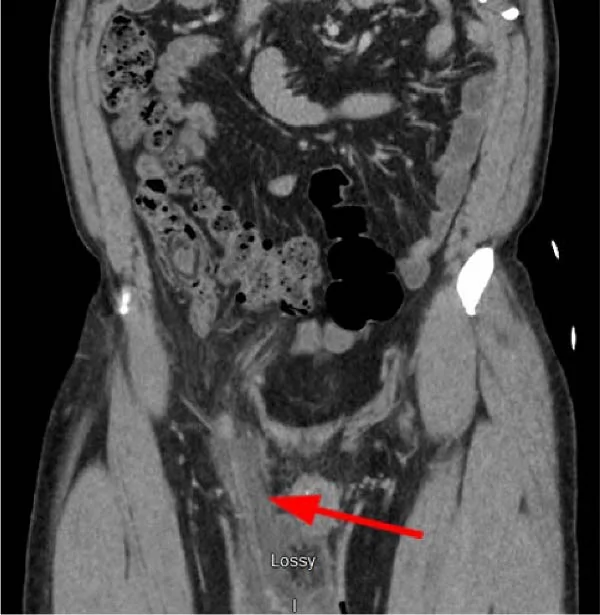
CT abdomen/pelvis. There is a fat‐containing hernia of the right inguinal region.
